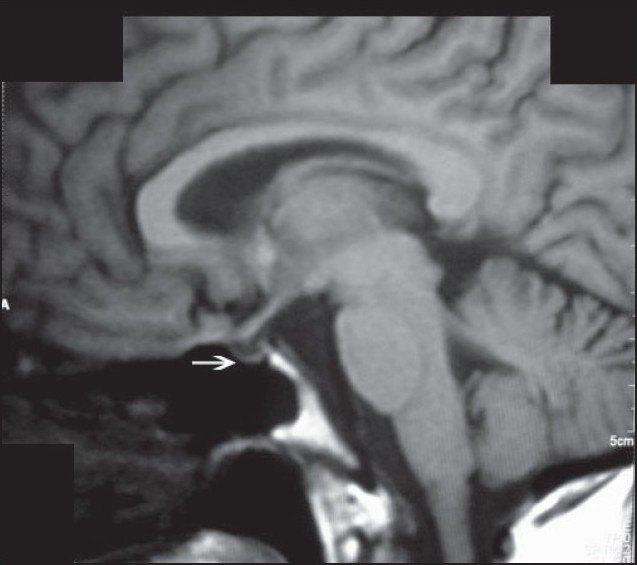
T1 weighted MRI pituitary, sagital view showing pituitary fossa filled with cerebrospinal fluid with stalk touching the base against a thin rim of compressed gland suggestive of an empty sella (arrow).
